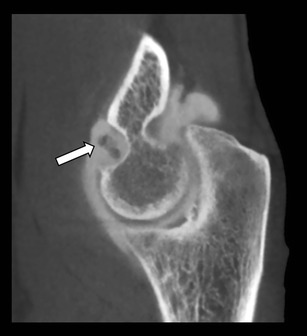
Intra-articular loose bodies. Sagittal CBCT-A reformatted image shows the presence of intra-articular loose bodies (arrow)
